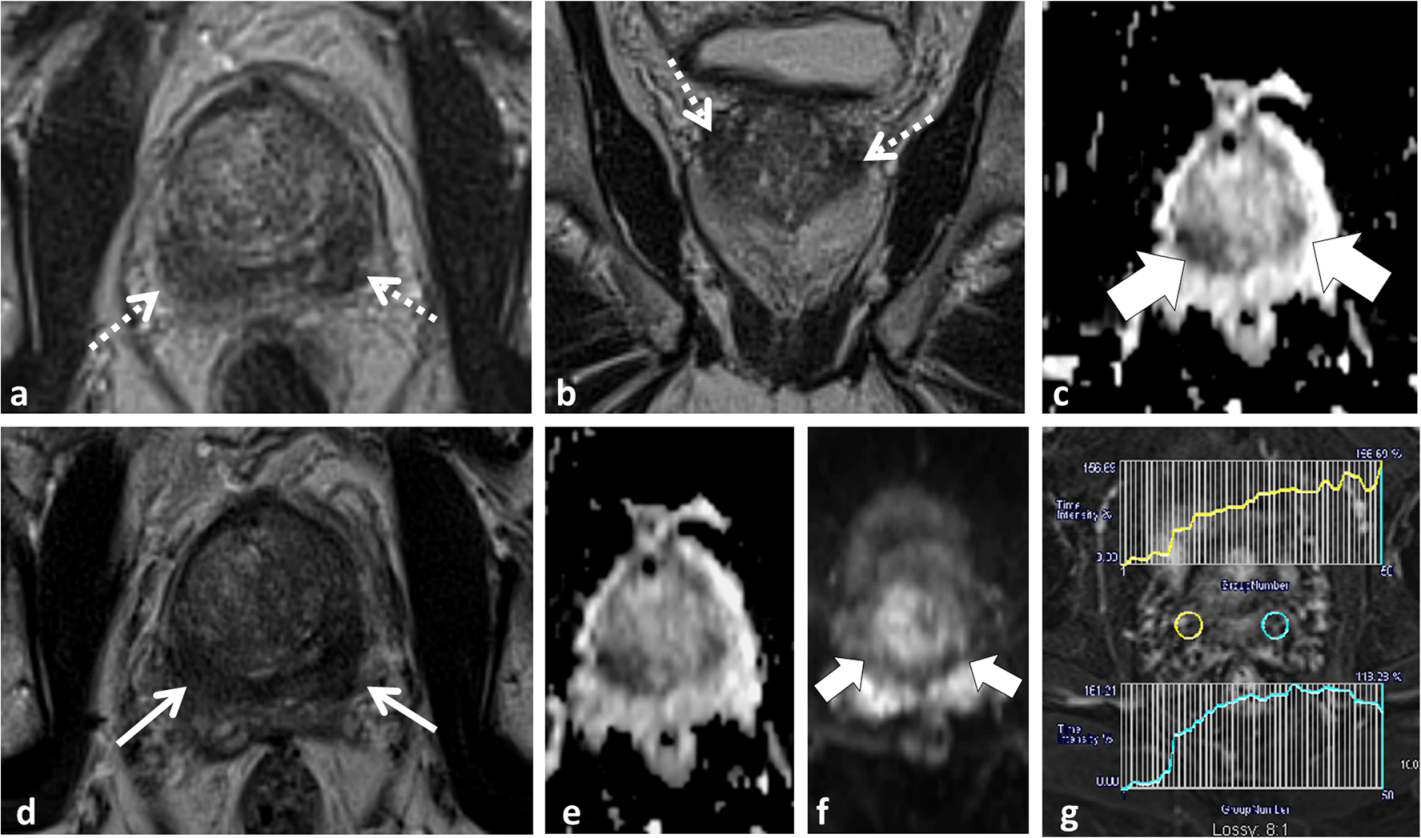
A 54-year-old male with low volume Gleason score 3 + 3 = 6 PCa at TRUS-guided biopsy in the right middle peripheral zone (*PZ*). MP-MRI was performed because of rising PSA to exclude clinically significant (*CS*) tumour. Axial (a) and coronal (b) T2-weighted (T2W) turbo spin echo (*TSE*) images demonstrate bilateral foci of low T2 signal intensity (SI) at the prostate base (*dotted arrows*) adjacent to the insertion of the vas deferens (not shown). Axial apparent diffusion coefficient (*ADC*) map (c) at the same level demonstrates corresponding low SI (*white arrows*). These areas were described as suspicious and repeat targeted TRUS-guided biopsy was suggested. A repeat biopsy with multiple cores through both areas yielded only normal prostatic tissue. A repeat MRI performed 2 years later demonstrates similar findings on the T2W (d) and ADC map (e), which represent the normal central zone. Corresponding b1000 mm²/s trace echo-planar image (EPI) does not show concordant areas of increased SI (*white arrows* in f) and there is benign progressive enhancement on dynamic contrast-enhanced (DCE) imaging (g)
